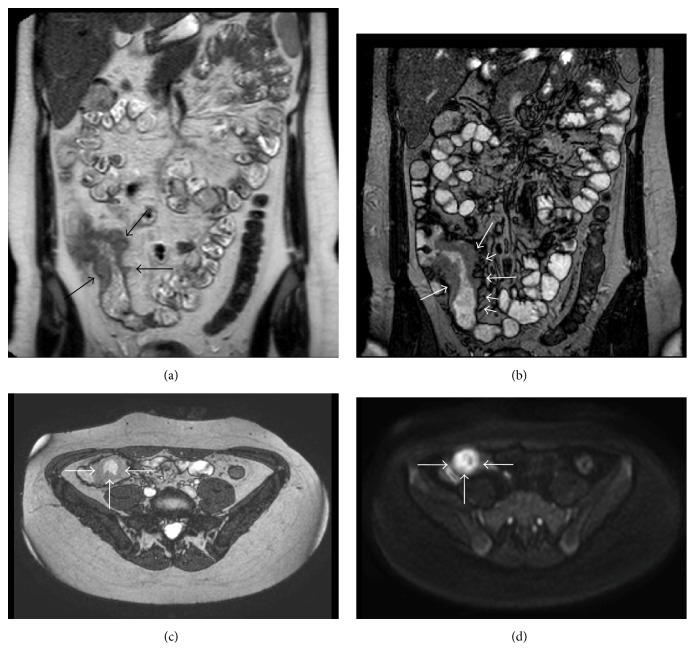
Diffuse bowel wall thickening with polypoid appearance* (long arrows)* and ectatic vasa recta* (short arrows)* are well demonstrable on coronal HASTE (a) and True-FISP (b) MR images as well as on axial True-FISP MR image (c). Axial diffusion-weighted MR image, performed at *b*-value of 800 s/mm^2^, shows restricted diffusion of water within the affected bowel wall (d).
